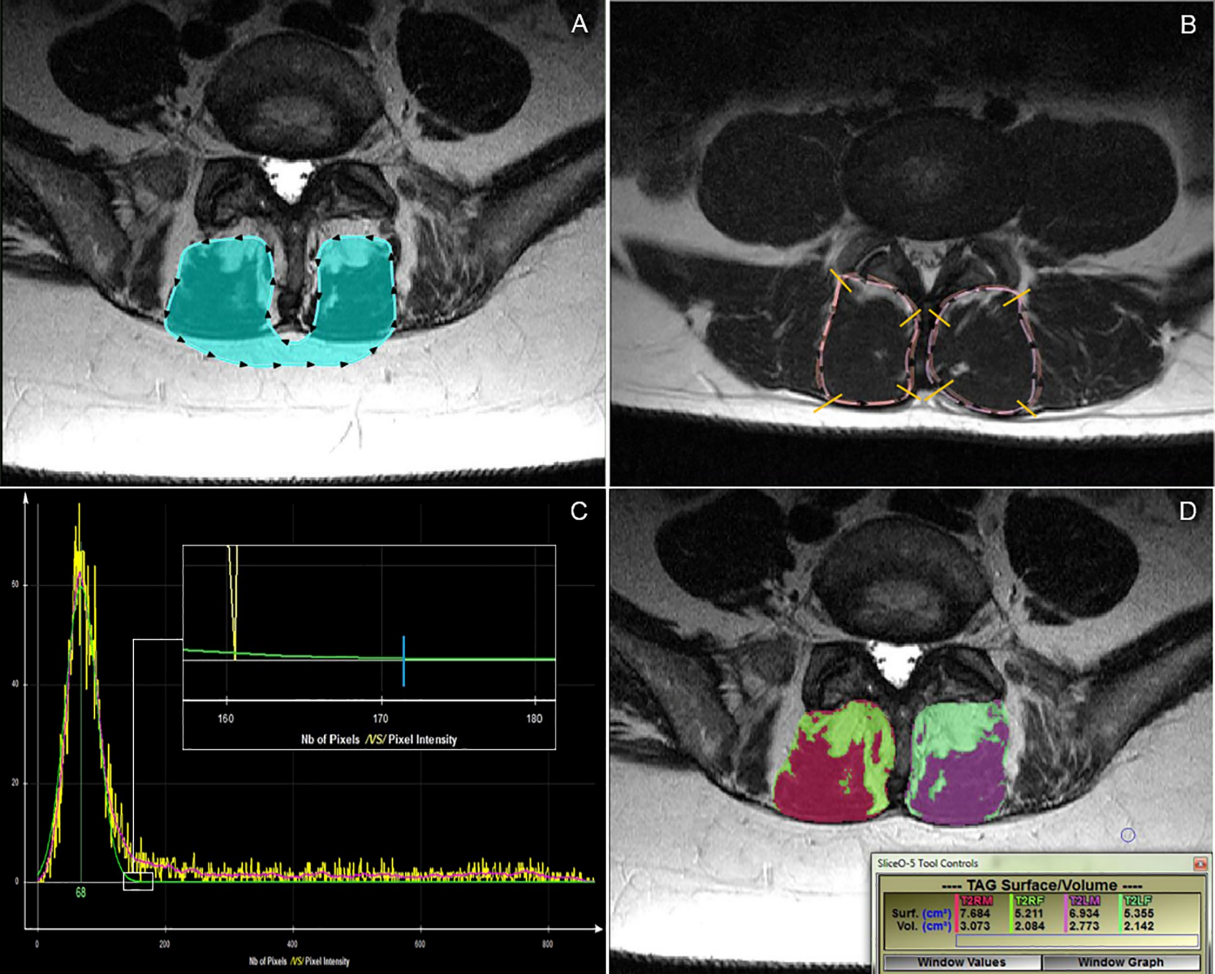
Different measurement procedure examples. A: Histogram outlining process. B: Histogram from A. Small white box indicates the region of intersection of the green histogram line with the X-axis at “0”. Note: to identify the point at which the histogram curve contacted the X-axis to the nearest value of 10, one must zoom in on the histogram within the sliceOmatic program. The inset box shows the point of intersection of the green histogram line with the X-axis, indicated by the blue line. In this example, the cut-off point would be 170. C: Overlapped T1-weighted and T2-weighted total cross-sectional area outlines for visual comparison. D: Fat (bright green/pale green) and muscle (red/purple) tissue highlighted, with related measurement outcomes [surf. measures (cm^2^) apply].
